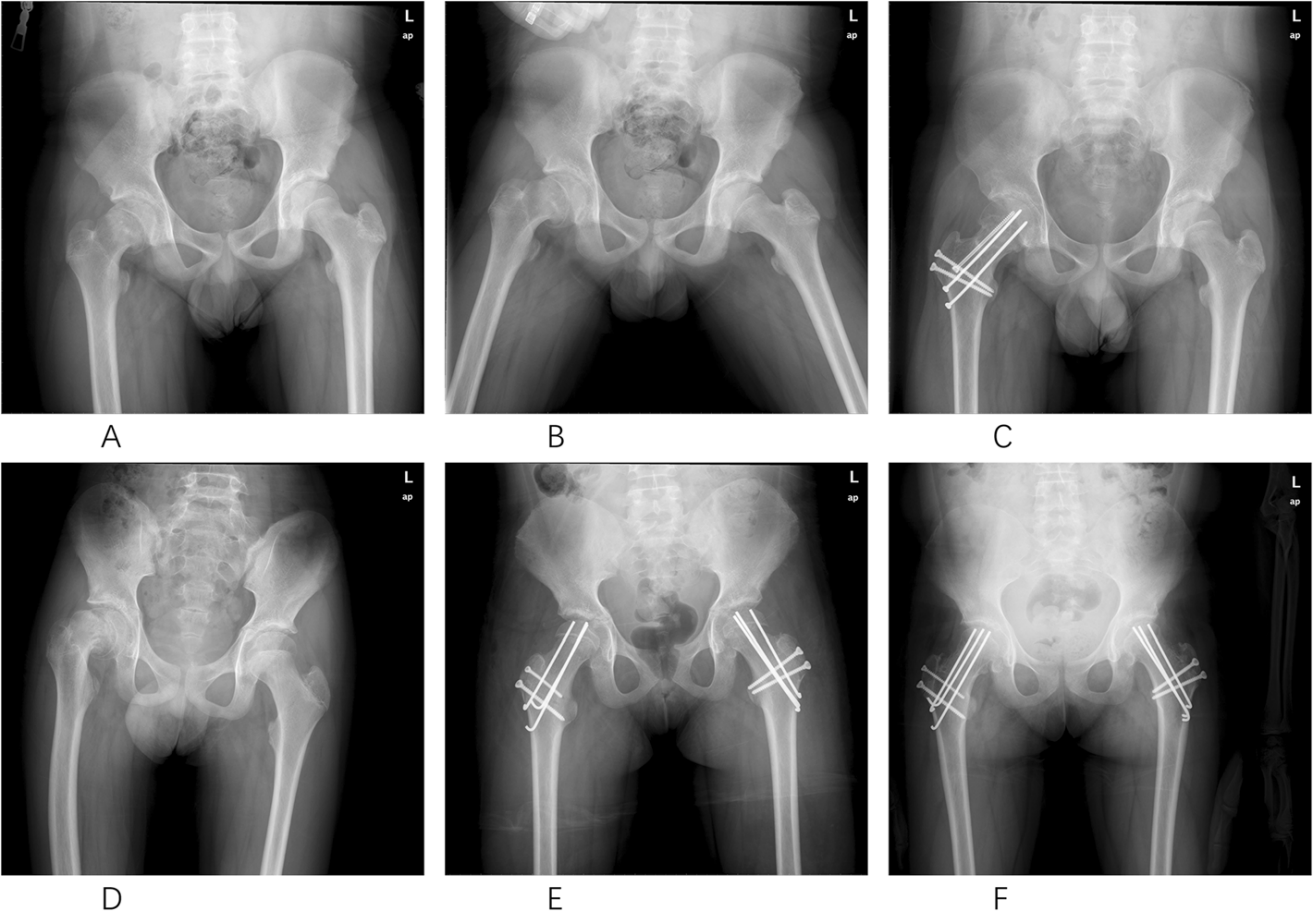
a–c A 10-year-old girl with severe pain and functional disability. a AP and b frog-leg lateral radiographs revealing a severe SCFE of the right hip. c AP radiographs obtained 2 years after surgery with no signs of AVN. AP indicates anteroposterior. d–f A 17-year-old girl with bilateral severe SCFE. d AP radiographs revealing severe SCFE of the bilateral hips. e AP radiographs obtained after the fixation. f AP radiographs obtained 2 years after surgery with no signs of AVN. AP indicates anteroposterior
